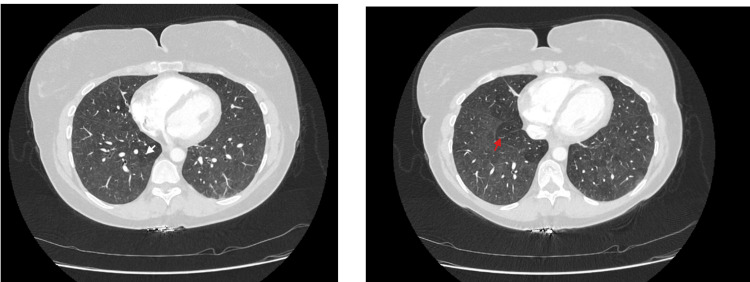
CT thorax with contrast while on vedolizumab therapy. White and red arrows indicate areas of air trapping.
